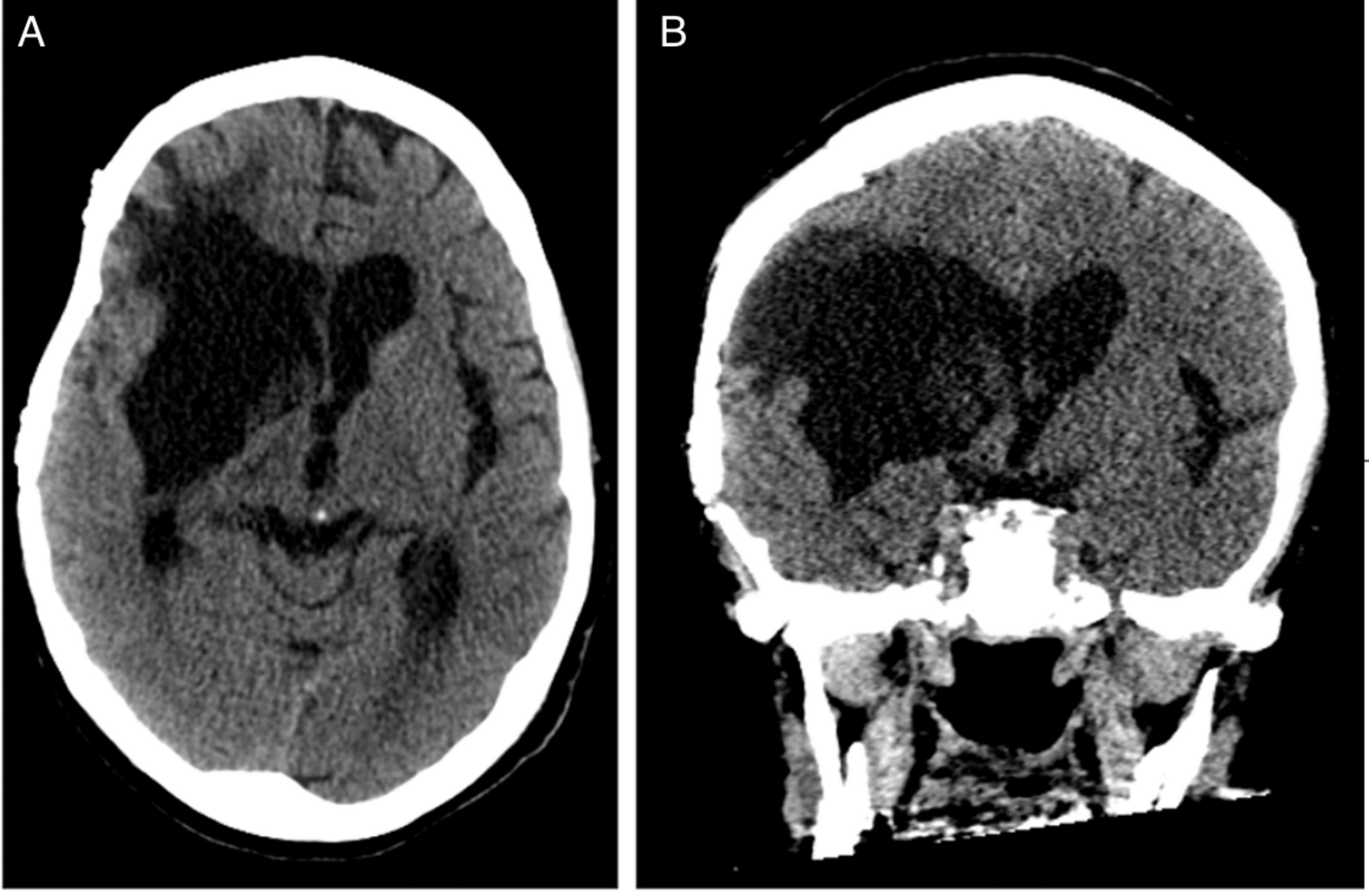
CT of the head performed 9 months following intraparenchymal hemorrhage. Axial (A) and coronal (B) images show encephalomalacia in the prior intraparenchymal hemorrhage bed with resolution of her midline shift following cranioplasty.
